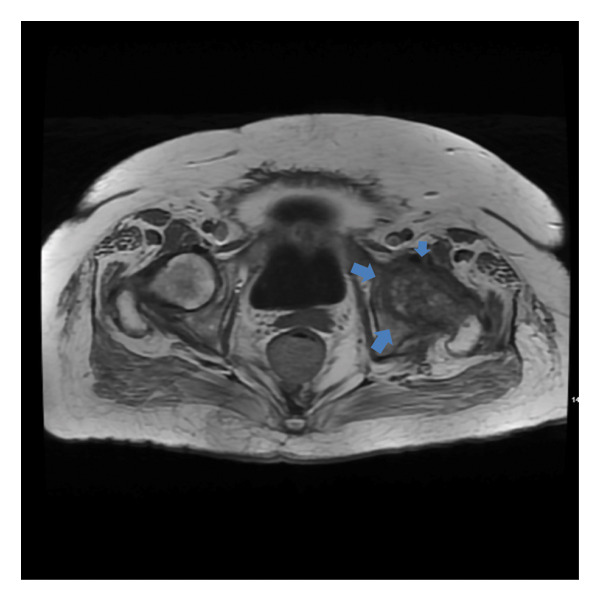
T1 Axial view on MRI of left hip with and without contrast showing patchy decreased T1 marrow signal (blue arrows) with increased T2 signal and enhancement centered within the left hip. Findings consistent with left hip septic arthritis and subjacent osteomyelitis.
